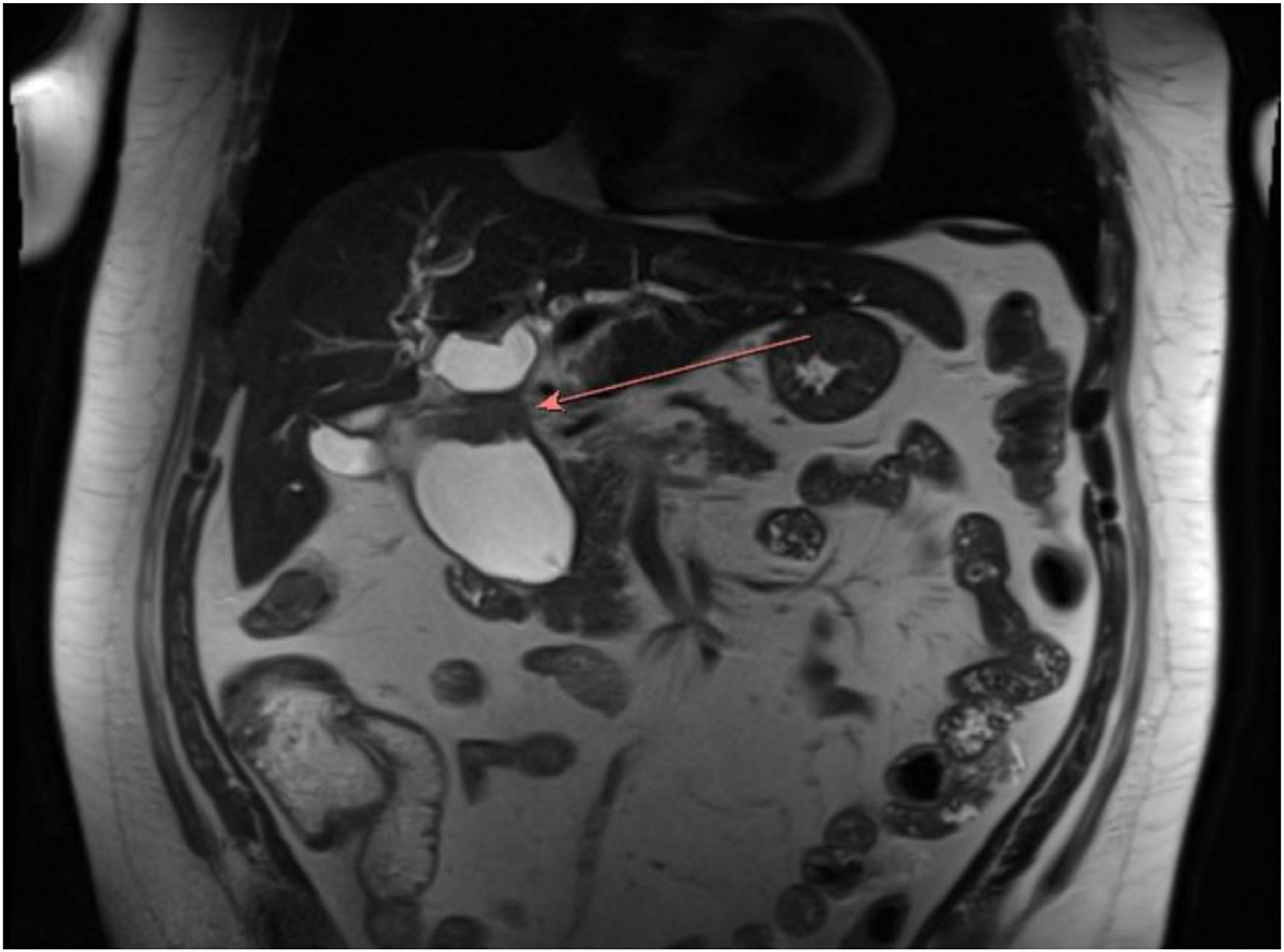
T2 weighted MRI/ MRCP coronal image of the upper abdomen without intravenous contrast shows marked abnormal dilation of the extrahepatic biliary tree suggestive of choledochal cyst. The arrow indicates focal, mass-like thickening of the distal common hepatic duct with associated stricture highly concerning for malignancy. Upstream dilation of the common hepatic duct and intrahepatic biliary tree is noted.
